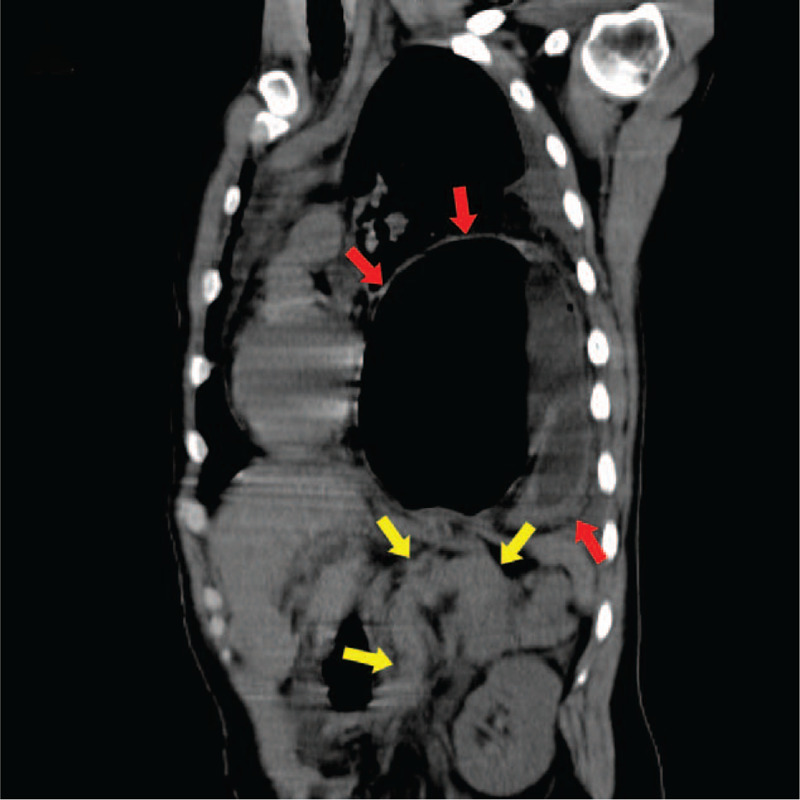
Axial CT image indicated intrathoracic stomach herniation through the left diaphragmatic defect (red arrows), meanwhile, bulky and folded into a “Ω” shaped pancreas closed to the diaphragmatic defect (yellow arrows). CT = computerized tomography.
